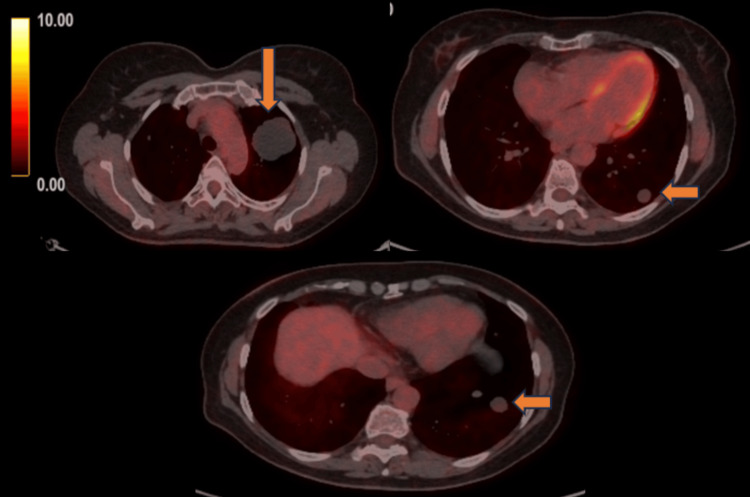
PET scan images obtained at the time of presentation show no visible FDG uptake in the multiple small lung nodules and masses (SUVmax 0.4–0.6) PET: positron emission tomography; FDG: fluorodeoxyglucose; SUVmax: maximum standardized uptake value
